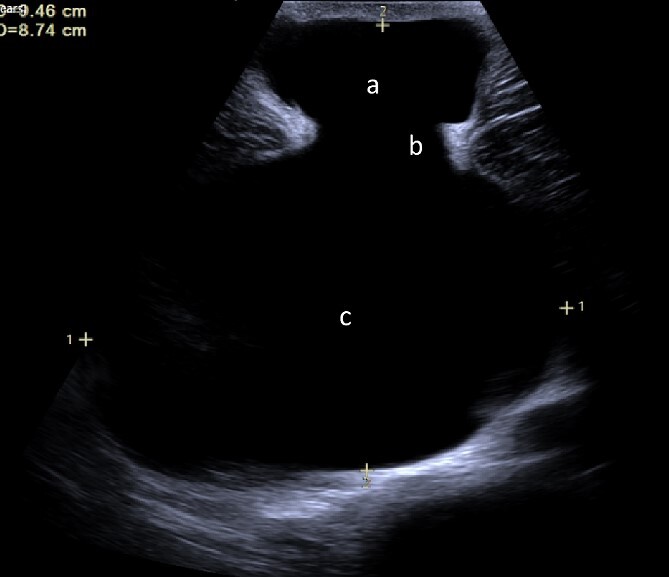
Ultrasound image demonstrating fluid collection within the umbilicus (a) tracking into abdominal cavity (c) originating from the cicatrix (b).
